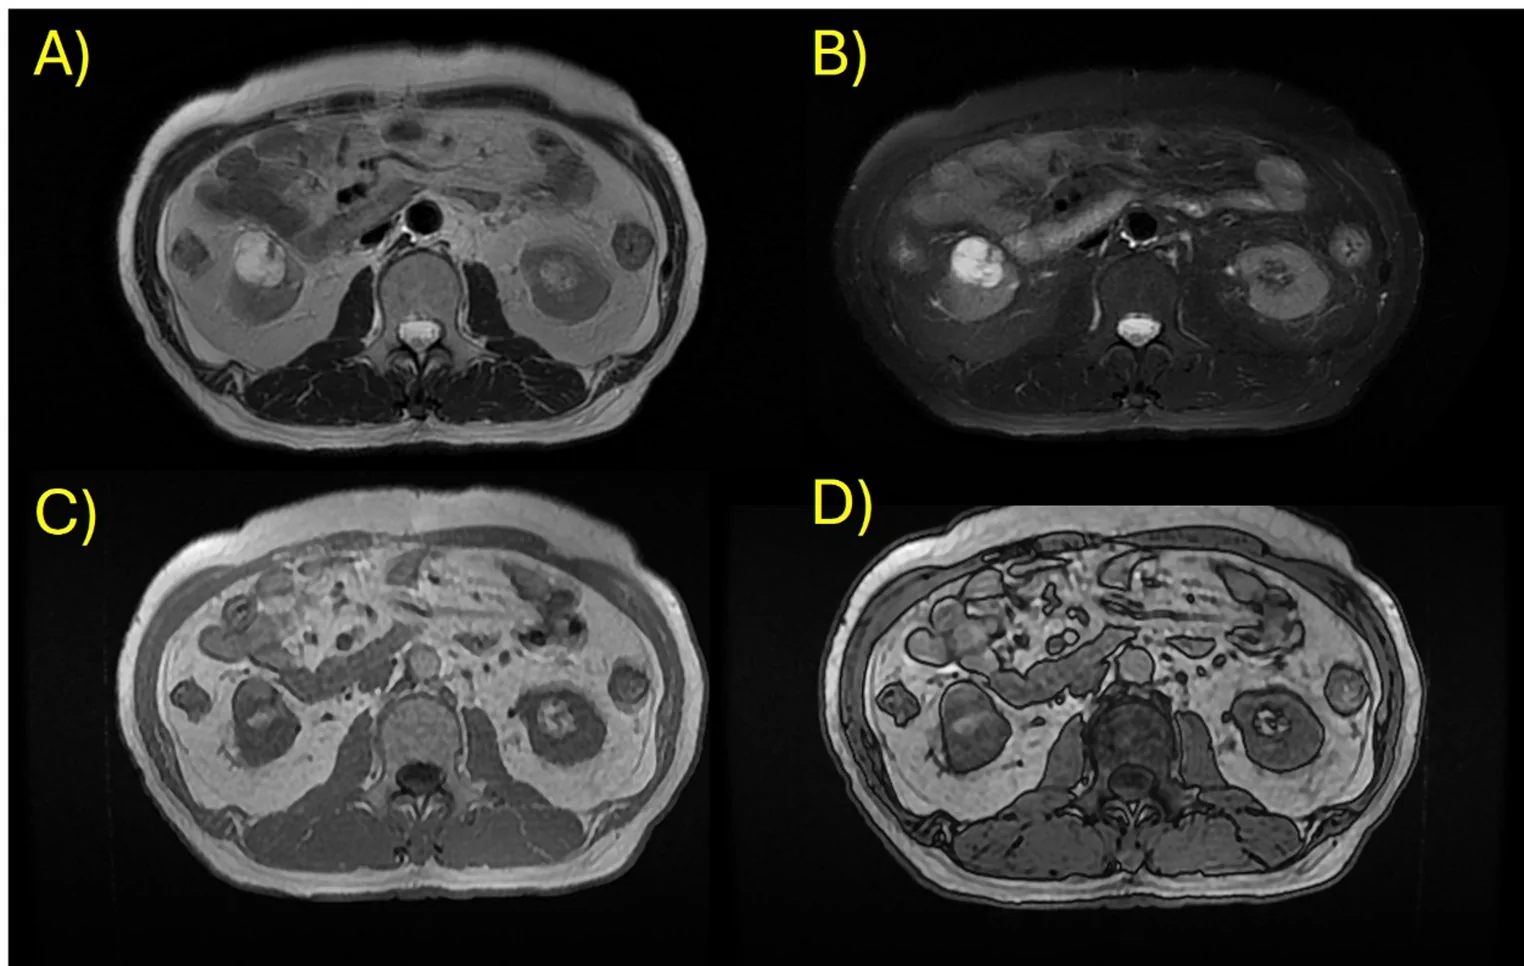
MRI images: axial T2-weighted sequences without (A) and with fat suppression (B) showed a complex cystic lesion of the right renal lower pole. In-phase and out-of-phase T1-weighted sequences (C and D) showed no signal drop, indicating absence of macroscopic fat
